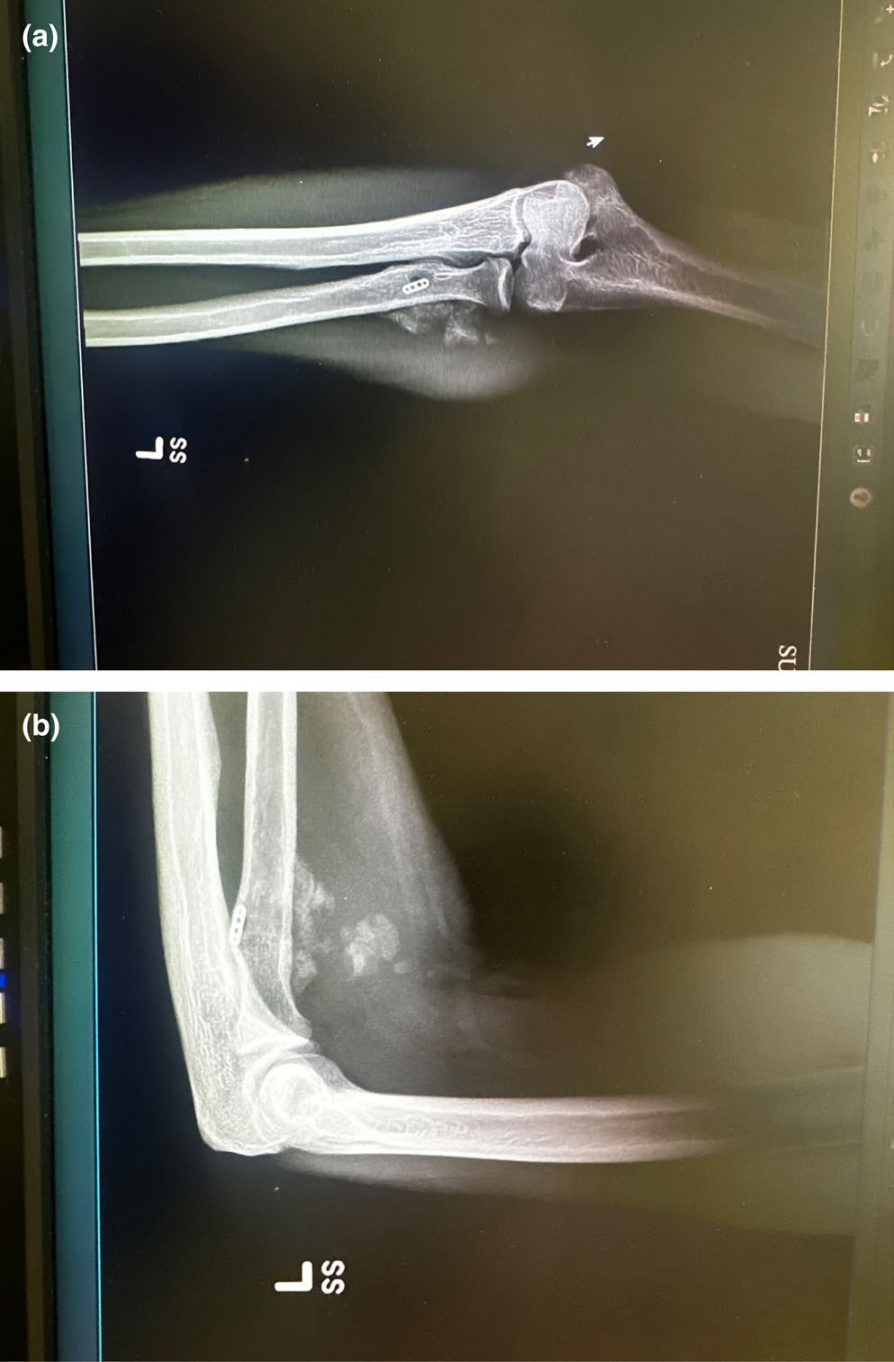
(a) Preoperative anterior to posterior radiograph of left elbow, demonstrating heterotopic ossification after primary repair of the distal biceps tendon. EndoButton is in an appropriate position sitting 3 × 2 × 3 cm within the radial tuberosity. (b) Preoperative lateral radiograph image of left distal bicep showing hetero ossification superior to the radial tuberosity. EndoButton is well aligned.
